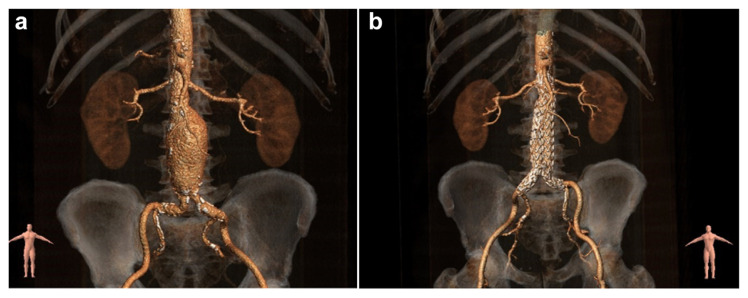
Three-dimensional computed tomography reconstruction of a 70 mm AAA before (panel A) and after (panel B) endovascular treatment.
